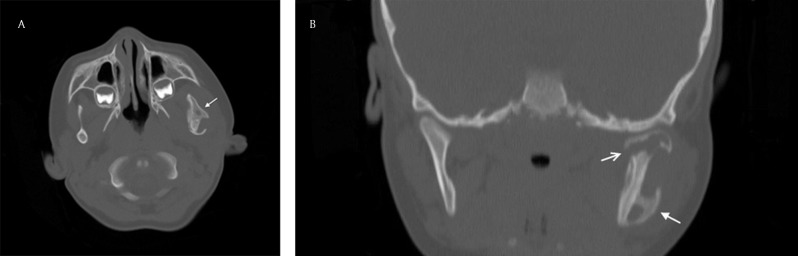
(A); Axial CT image of the mandible in bone window settings showing a sclerotic thickening of the left posterolateral portion of the mandible with parallel periosteal reaction (solid white arrow); (B) Coronal reformatted CT image of the mandible in bone window settings showing a sclerotic thickening of the left posterolateral portion of the mandible with parallel periosteal reaction (solid white arrow) and a cortical disruption of the left mandibular head (hollow white arrow).
